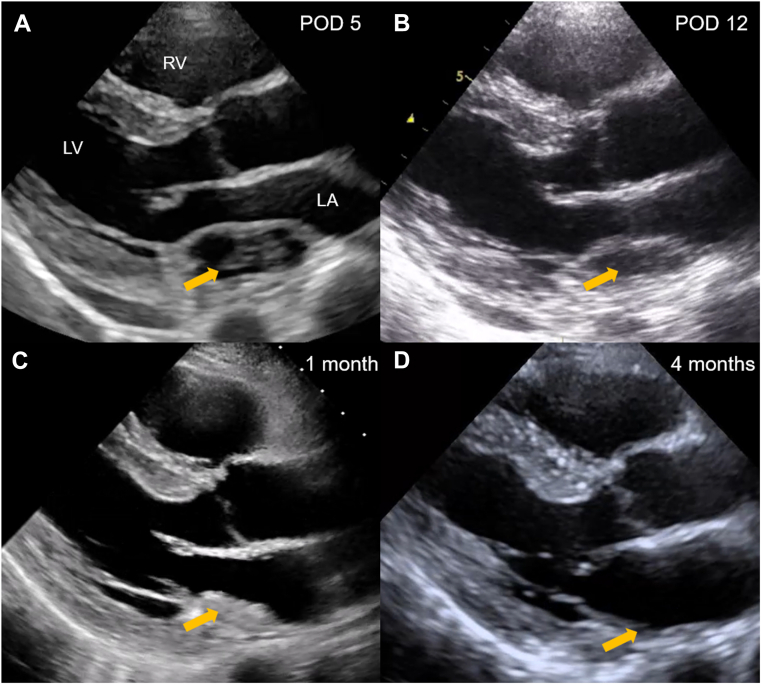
Serial Transthoracic Echocardiography Images TTE performed on (A) postoperative day 5 and (B) postoperative day 12. A slight re-expansion occurred on postoperative day 5, subsequently demonstrating continued gradual regression in hematoma size (arrow). TTE images at (C) 1 month and (D) 4 months after discharge. LAIH demonstrated continued gradual regression, with near-complete resolution observed 4 months after discharge (arrow). POD = postoperative day; TTE = transthoracic echocardiography; other abbreviations as in Figure 4.
